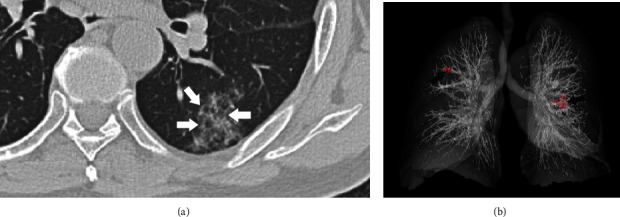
A 76-year-old man presented without onset symptoms. Axial CT image shows patchy ground-glass opacities located at the lateral basal segment of the left lower lobe (a). The interlobular septa (white arrow) are thickened and show a reticular pattern. The 3D reconstruction image shows bilateral opacities (red spot and black arrow) with peripheral distribution (b).
